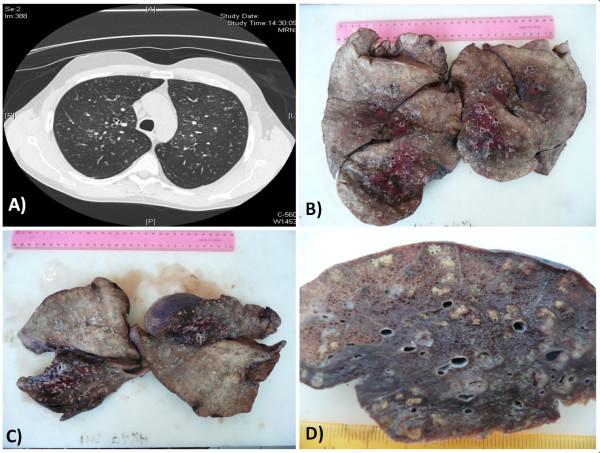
Chest HRCT scan and gross examination of the lungs. (A) HRCT scan: nodular shadows were distributed in a centrilobular fashion, often extending to small branching linear areas of attenuation (“tree-in-bud” pattern); (B-D) gross examination of the lungs showed bilateral multiple white-yellowish small nodules centred on small airways.
